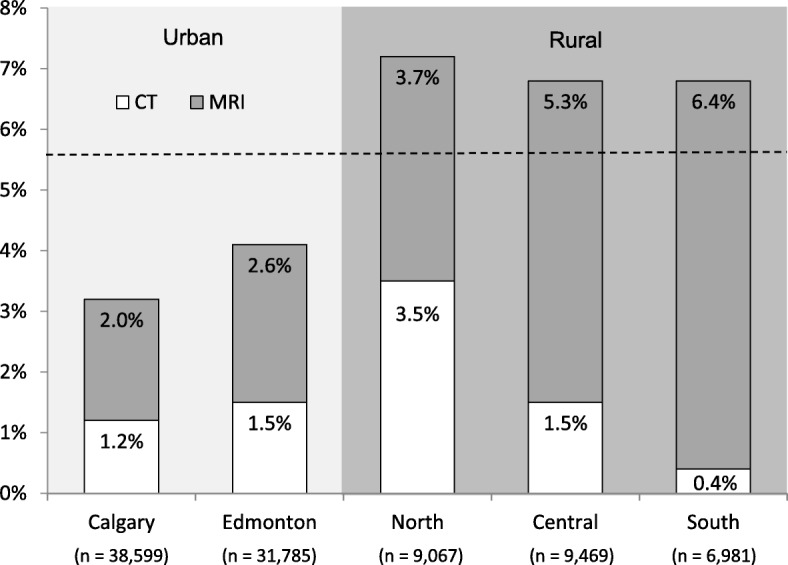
Risk-adjusted CT and MRI scan rates at 6 months after index visit for lower back pain by Albertan health zone
